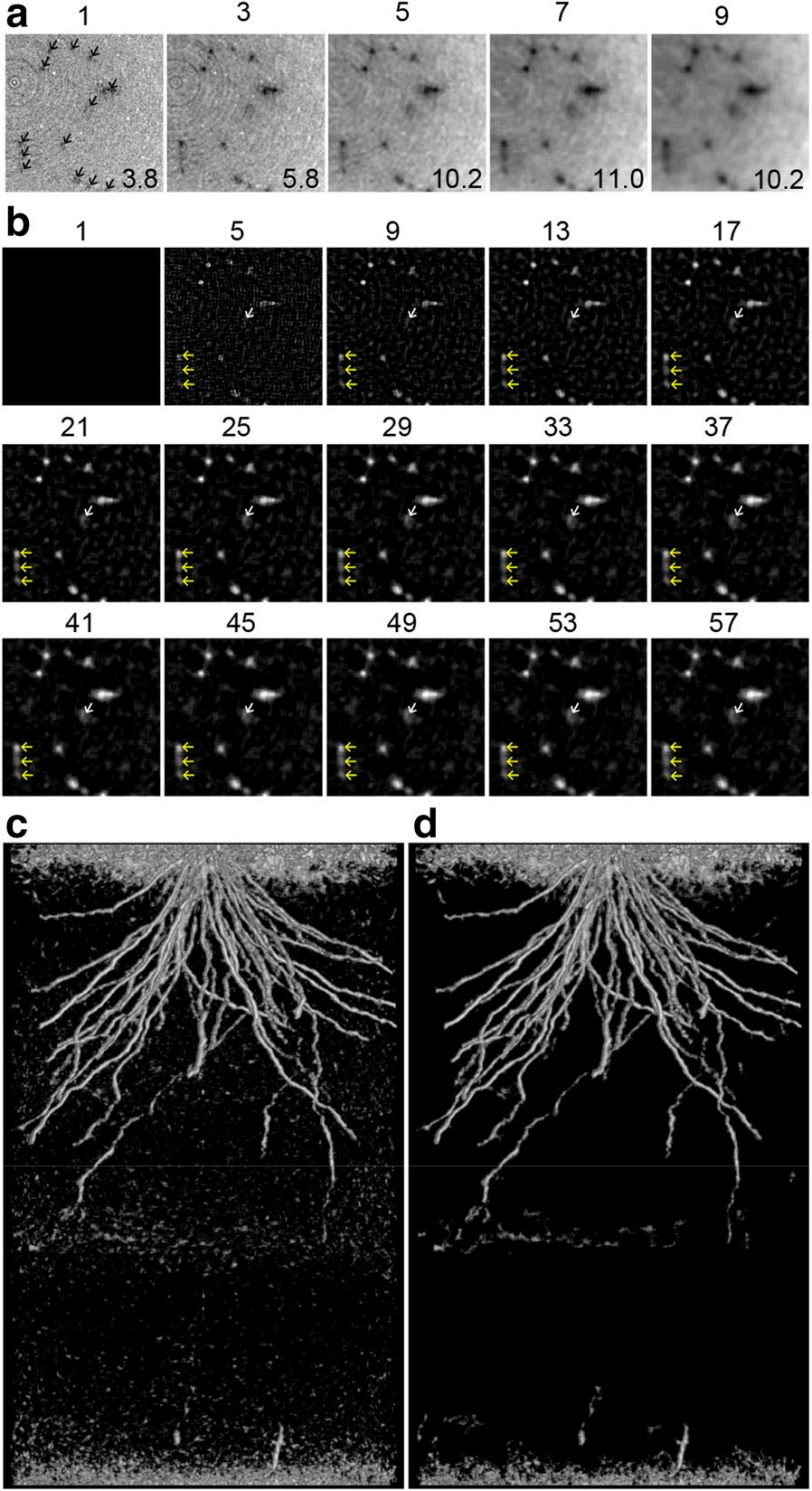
X-ray CT volume image processing, using a 4-week-old Kinandang Patong as the scanning subject: a scaled-up horizontal slices of an X-ray CT volume, filtered using 3-D median filters of kernel sizes 1, 3, 5, 7, and 9, with the numbers above the images indicating kernel size, and numbers on the images indicating the contrast-to-noise ratio. Arrowheads in the leftmost image indicate representative root fragments; b effect of blur filter kernel size on edge detection. Numbers above the images indicate the kernel size, representative root fragments are indicated by arrowheads, roots indicated by the white arrowhead are hardly visible using kernel size 5, and the roots indicated by the yellow arrowheads appear stuck together when using a large kernel size, such as 57; c horizontal projection of the image-processed CT volume without thresholding or size opening; d horizontal projection of the image-processed CT volume with thresholding and size opening
